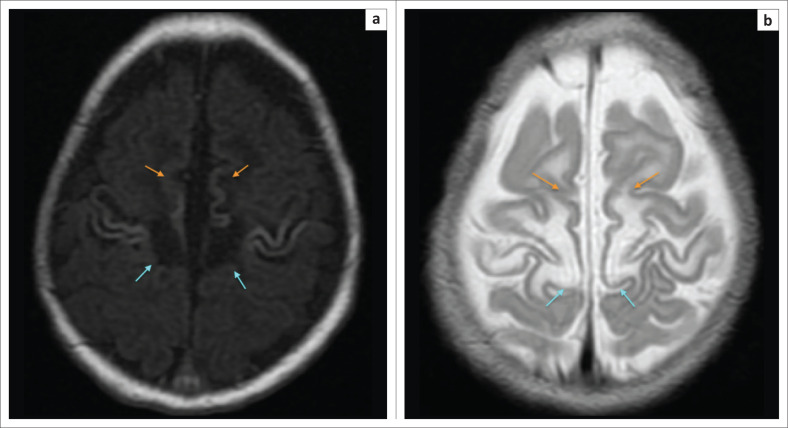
The same child as in Figure 6. Axial magnetic resonance imaging at the superior cerebral convexity demonstrating perirolandic cortical ribbon hyperintensity with T1 shortening (a) on both sides of the central sulcus and T2-weighted hyperintensity of the surrounding sensorimotor cortex (b). Note associated localised parasagittal cortex (orange arrows) and paracentral lobule (cyanide arrows) involvement.
